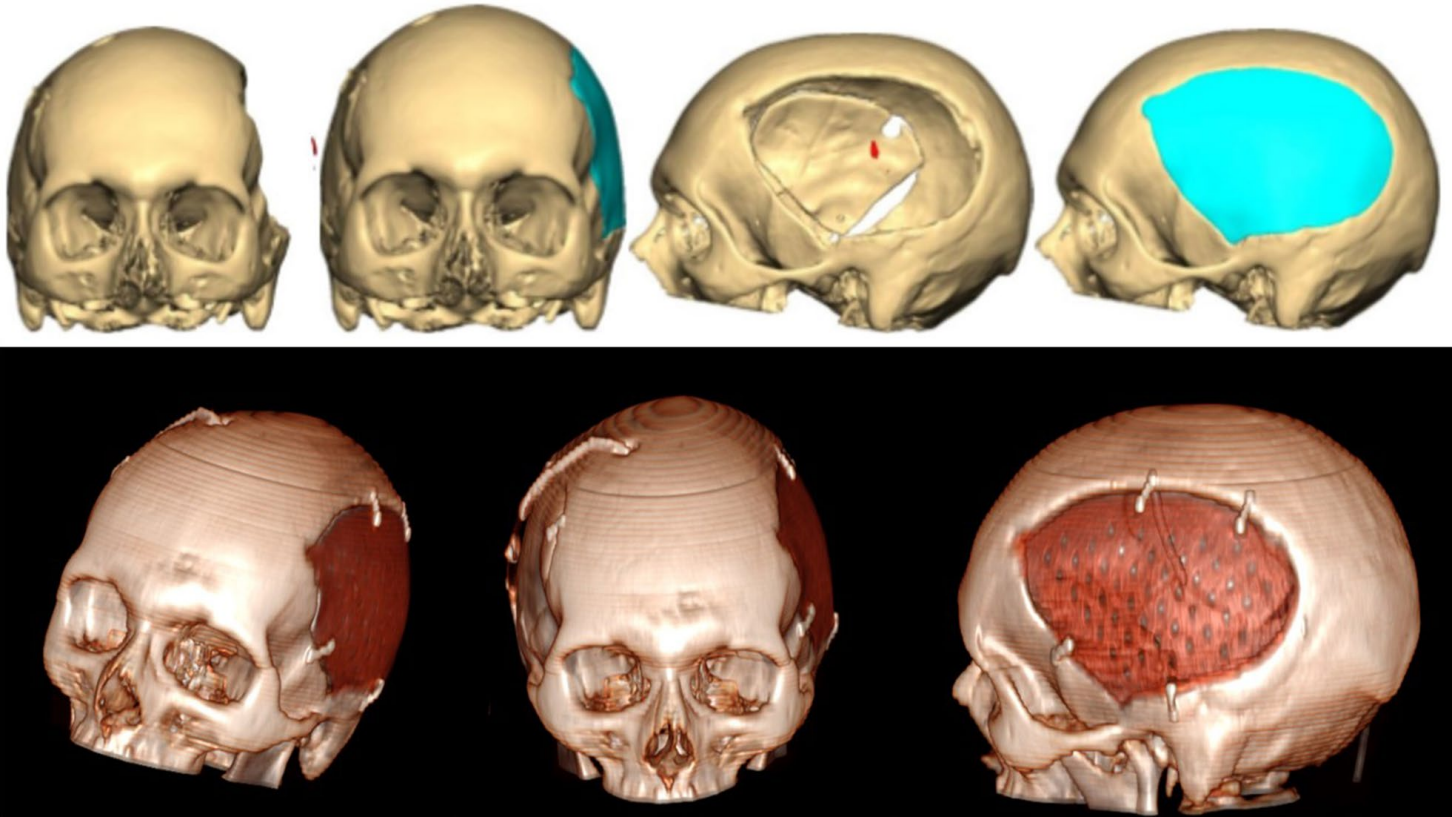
Tridimensional rendering of implanted PEEK cranioplasty. The relative “permeability” of PEEK to echoes allows for high quality intracranial ultrasound evaluation. PEEK, polyetheretherketone
